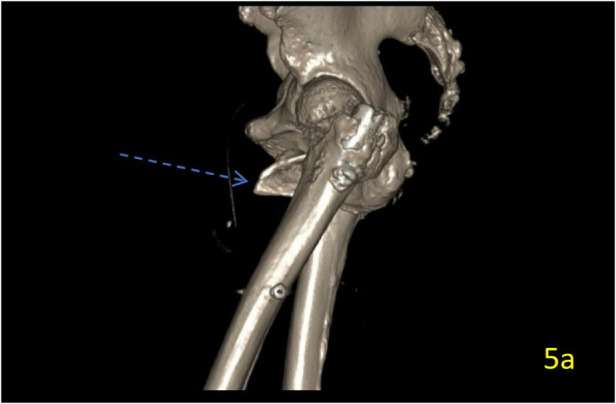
Lateral view of a postoperative 3D-CT scan of the left hip. Comparison with preoperative imaging confirms the resection of the previously displaced lesser trochanteric spike.
